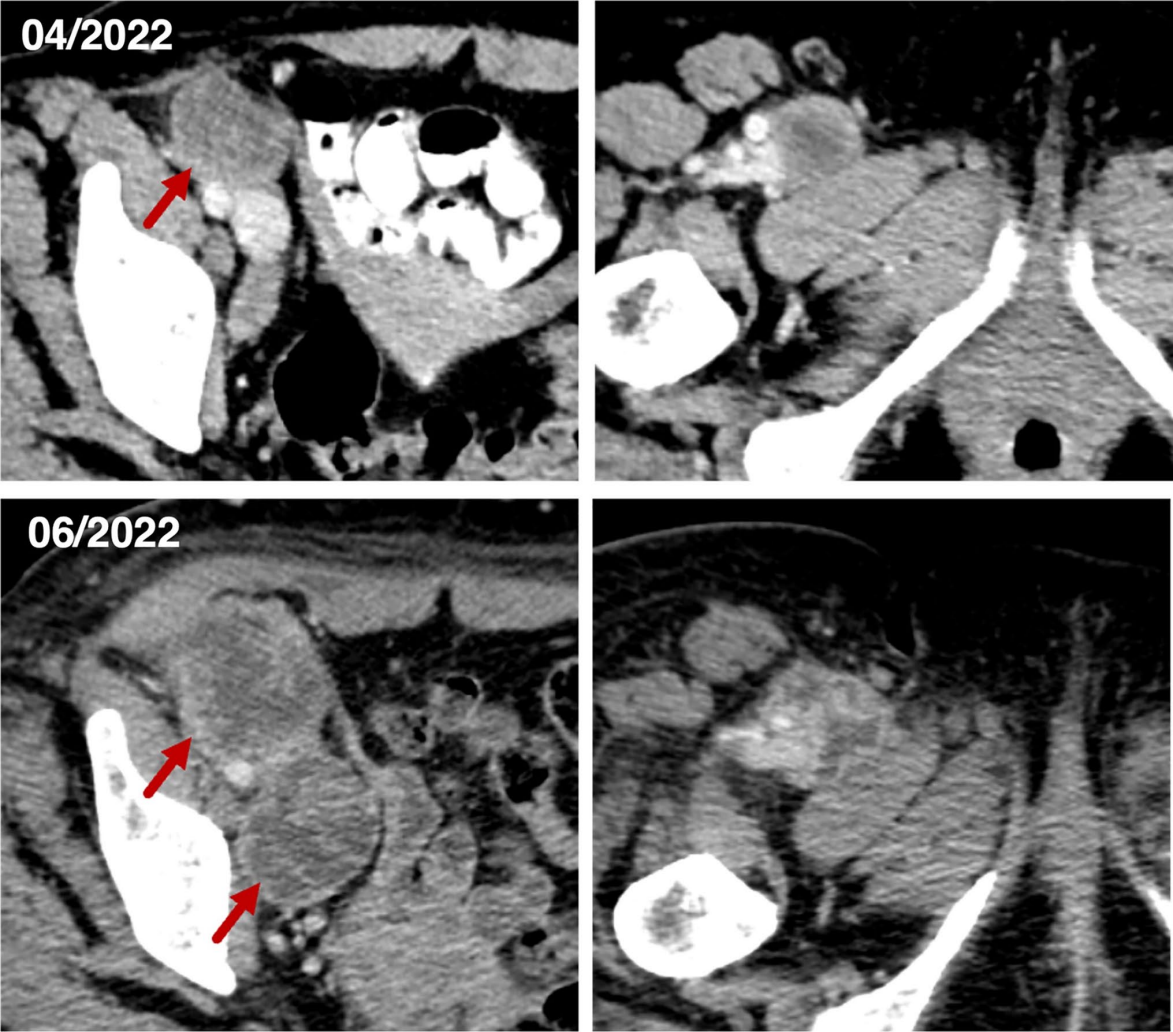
CT staging imaging of a 59-year-old female patient with an undifferentiated pleomorphic sarcoma in the right thigh region and metastasis to iliac and inguinal lymph nodes at baseline (04/2022) and after two cycles with doxorubicin/ifosfamide (06/2022). A central necrosis was identified in the detected metastatic lymph nodes at both dates. Besides a size progression, new suspicious lymph nodes occurred in follow-up imaging
